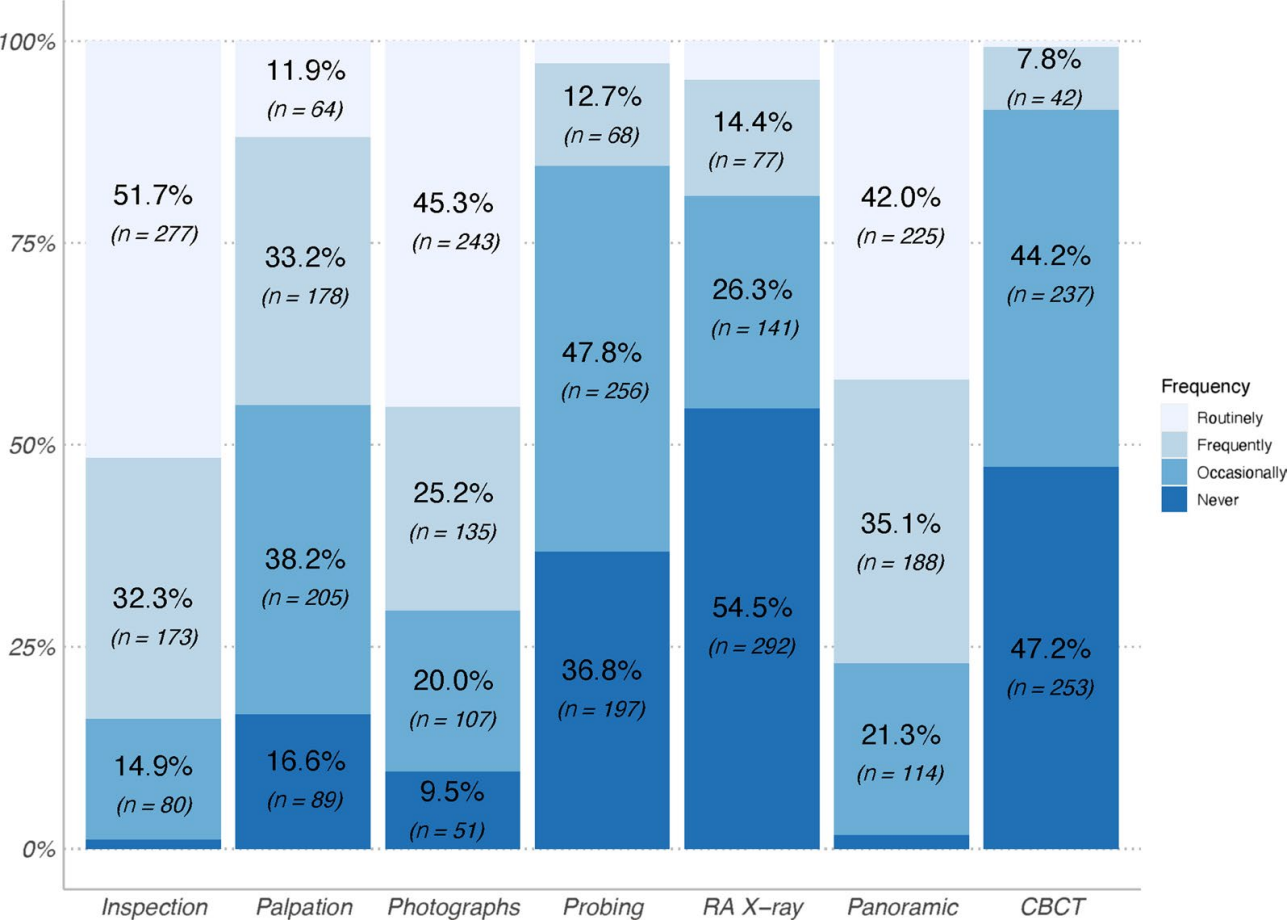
Frequency of use of different screening methods during orthodontic treatment by orthodontists. RA X-ray: retro-alveolar radiography, CBCT: cone beam computed tomography
